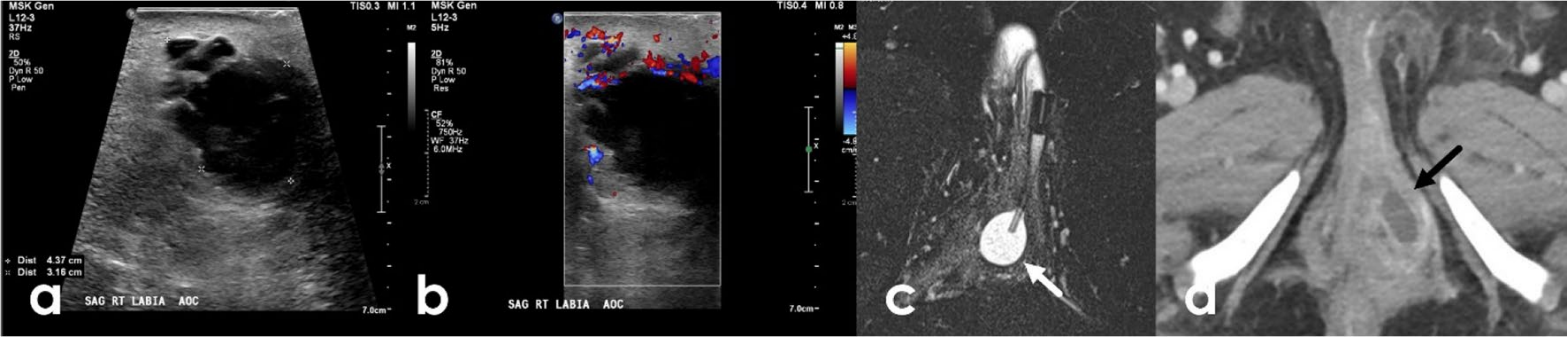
a–c. 26-year-old female with Bartholin gland abscess. Greyscale and color US images of the region of the Bartholin gland demonstrate a complex collection with surrounding hyperemia. Axial high res T2FS demonstrates a Bartholin gland abscess after placement of Word catheter (white arrow). d 85-year-old female with Bartholin gland abscess. Axial contrast enhanced CT images demonstrate a large rim enhancing collection in the area of the Bartholin gland with surrounding tissue stranding (black arrow)
